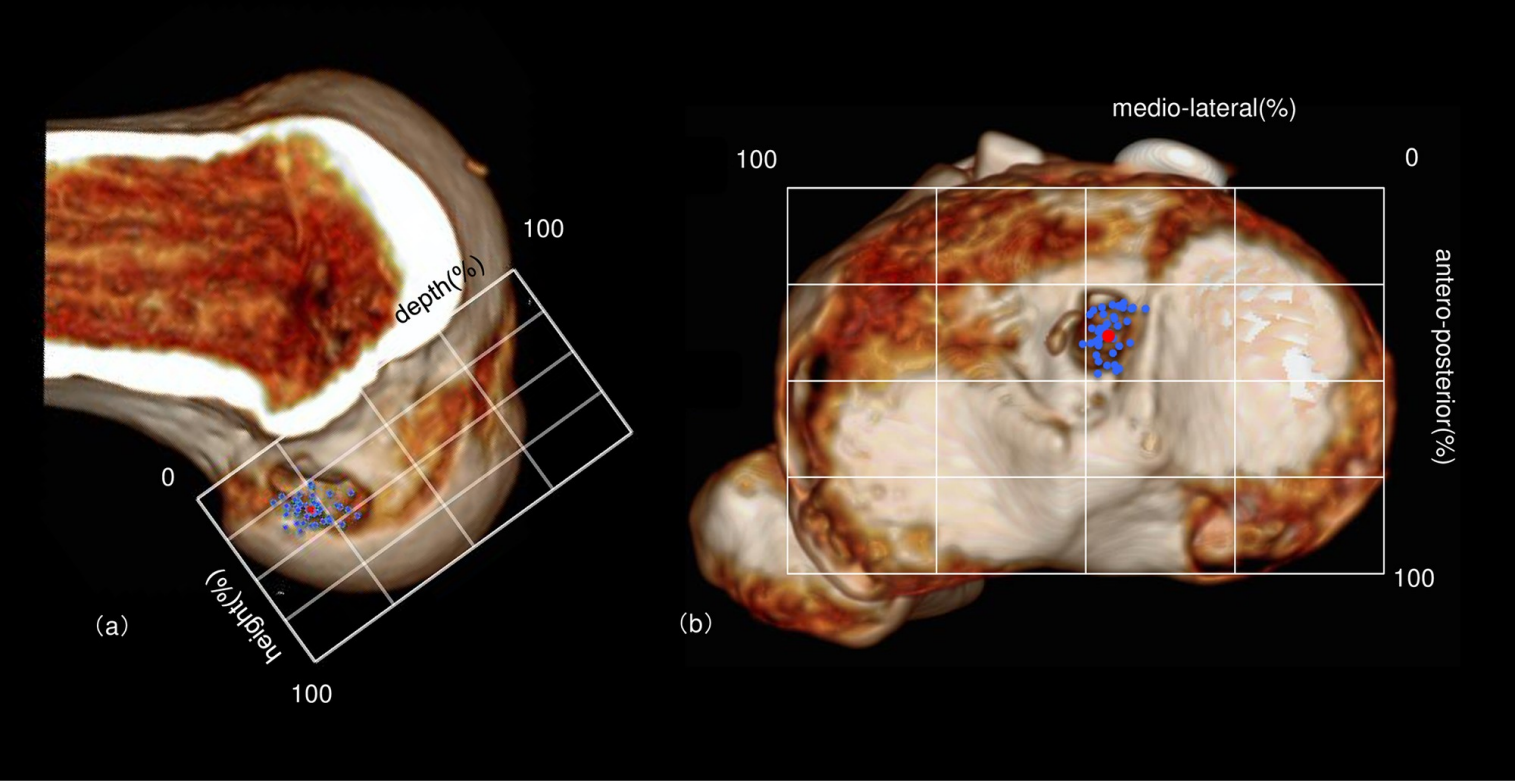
Evaluation of bone aperture positions using the quadrant method on 3-D CT scans. Evaluation of bone aperture positions using the quadrant method on 3-D CT scans taken 3 weeks after the operation. The analysis showed that all bone apertures were placed in anatomically appropriate positions. (a): femur, (b): tibia.
